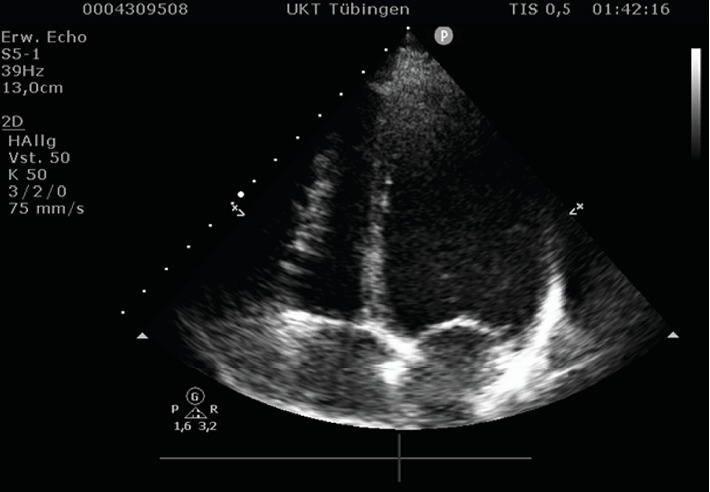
Echocardiogram, four-chamber view: extrinsic compression of the right ventricle.
